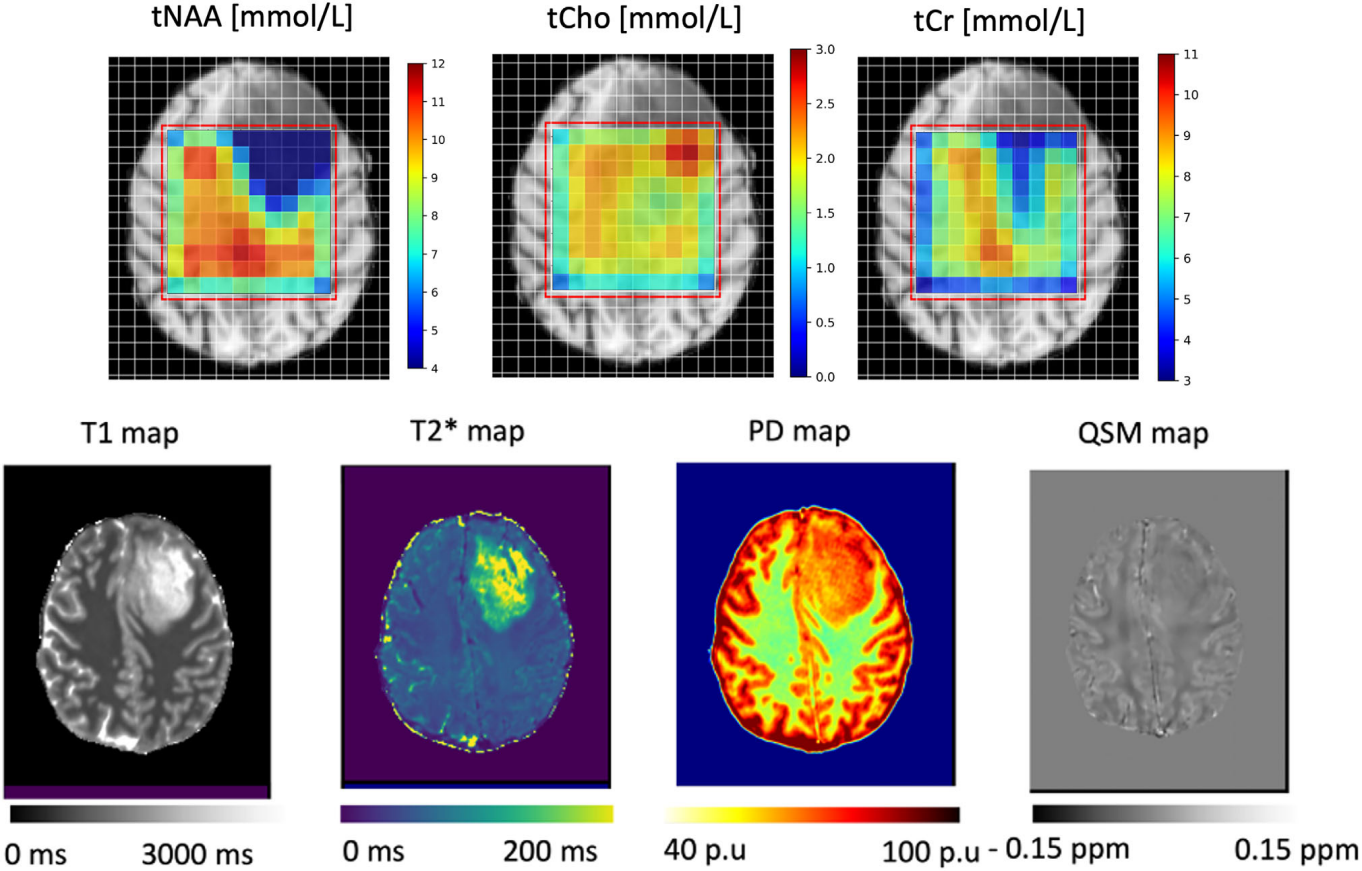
Final outputs of the absolute quantification approach using the Proposed‐method. *Upper row*: Metabolite concentration maps with individual‐specific correction for water‐relaxation effects (“tNAA,” “tCho,” and “tCr” metabolite maps are shown). *Bottom row*: The four quantitative MRI (qMRI) maps (T_1_, T_2_*, H_2_O, and quantitative susceptibility mapping [QSM]) obtained with the qMRI protocol are displayed. PD, proton density; tCho, total choline; tCr, total creatine; tNAA, total N‐acetylaspartate.
